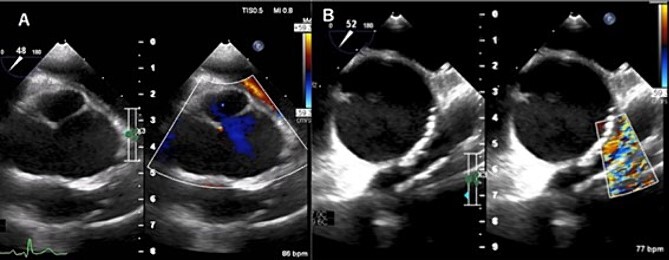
Transesophageal echocardiogram captured preoperatively shows a dilated sinus of Valsalva and mild central aortic valve regurgitation (A), and severe RVOT stenosis (B).
